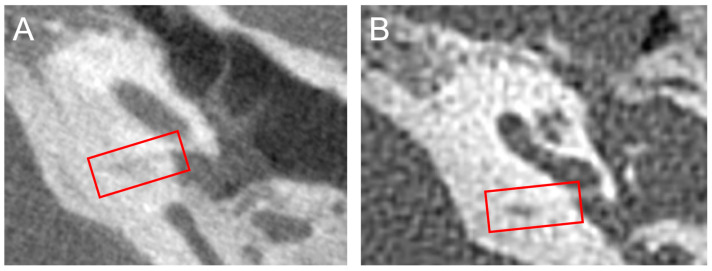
Side-by-side flat-panel and high-resolution computerized tomography of the same patient. (A) Flat panel CT scan (parameters described in the Methods section) demonstrating the improved resolution of the imaging modality as compared to the high-resolution CT. A red box encloses the cochlear aqueduct visible in the slice. The poor definition of the walls of the cochlear aqueduct is also seen. (B) High-resolution conventional CT scan (120 kV, 240 mV, collimation slice thickness 0.6 mm) of the same ear shown in Panel A. The generally poor resolution of the temporal bone and CA as compared to the flat-panel CT is evident. A red box encloses the cochlear aqueduct visible in the slice.
